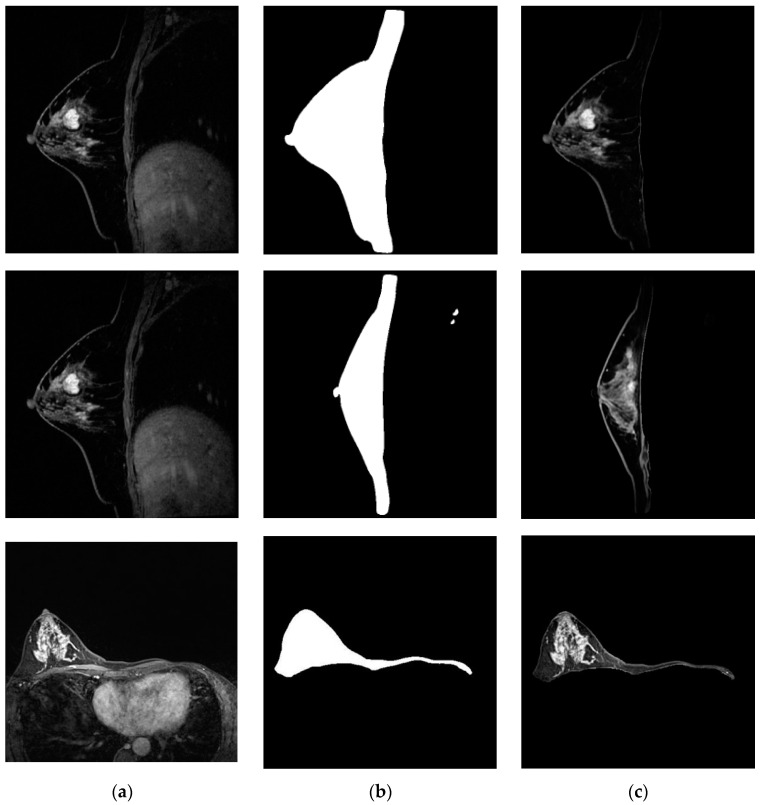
U-Net++ breast edge segmented: (a) sagittal breast MRI and single breast MRI at axial plane; (b) masks; (c) segmented breasts.
